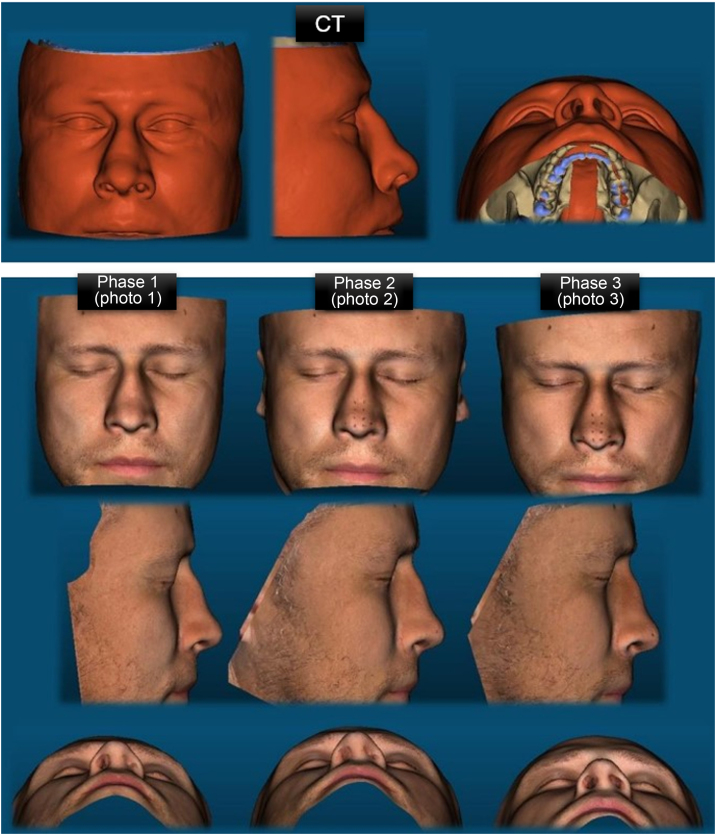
Representation of the 3D meshes produced in a male patient, reconstructed from the CT of the facial sinuses and by photogrammetry in the different capture phases.
